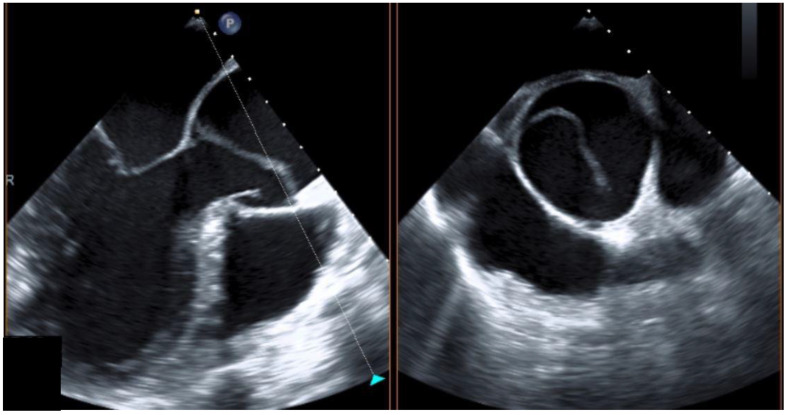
Transesophageal echocardiography in a pregnant woman with acute aortic dissection type A in the 38th gestational week.
